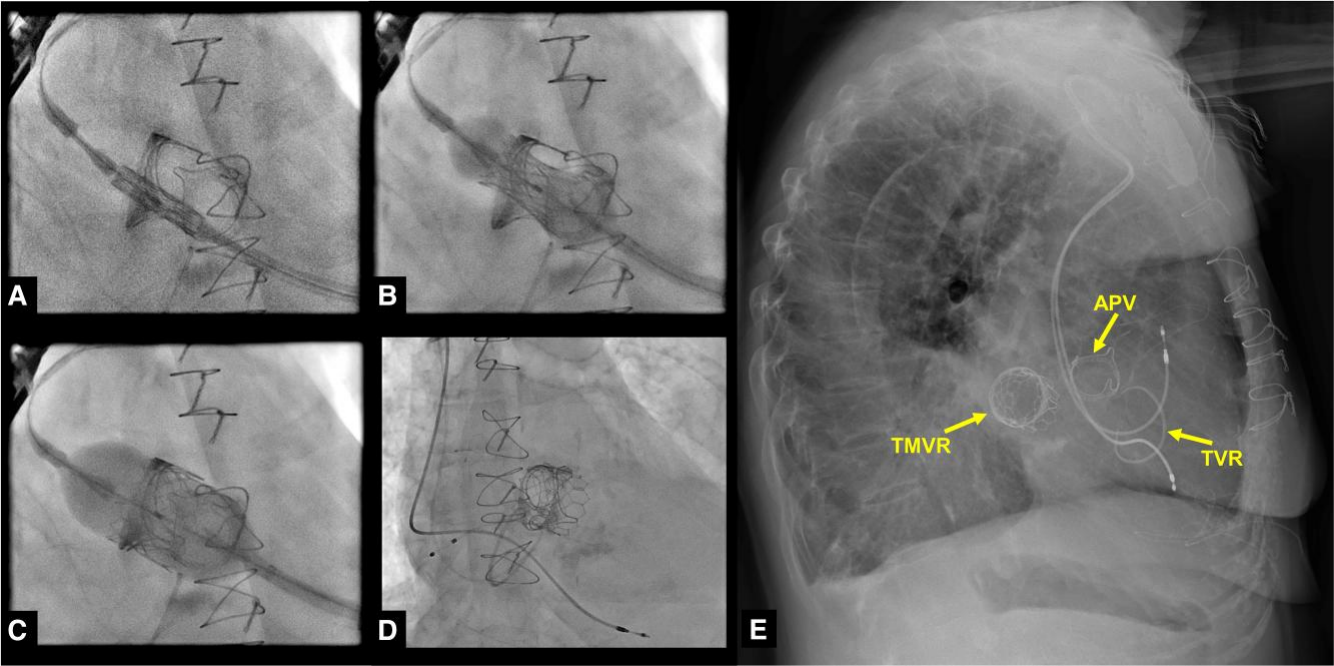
Several frames of the intraprocedural radioscopy depicting the slow and complete transapical placement of the 26 mm Sapien Ultra valve (Edwards Lifesciences, Irvine, CA) in the old mitral valve prosthesis (*A–D*). Lateral radiography showing the contours of the prosthetic aortic valve, tricuspid valve ring, and newly implanted Sapien Ultra valve as a ‘valve in valve’ in the mitral position (*E*). APV, prosthetic aortic valve; TVR, tricuspid valve ring; TMVR, transcatheter MV replacement.
